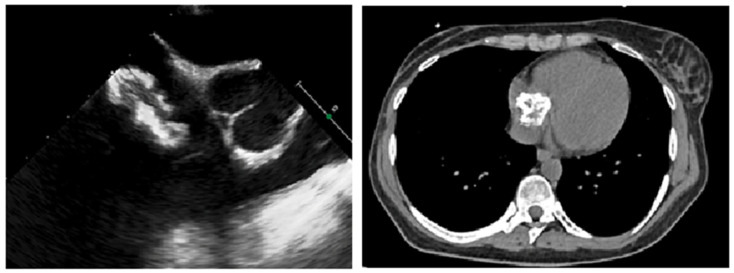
Case 2. (Left) TEE shows the calcified amorphous tumor (CAT) in the right atrium; (Right) axial contrast-enhanced CT angiography view showing the right atrial mass.
